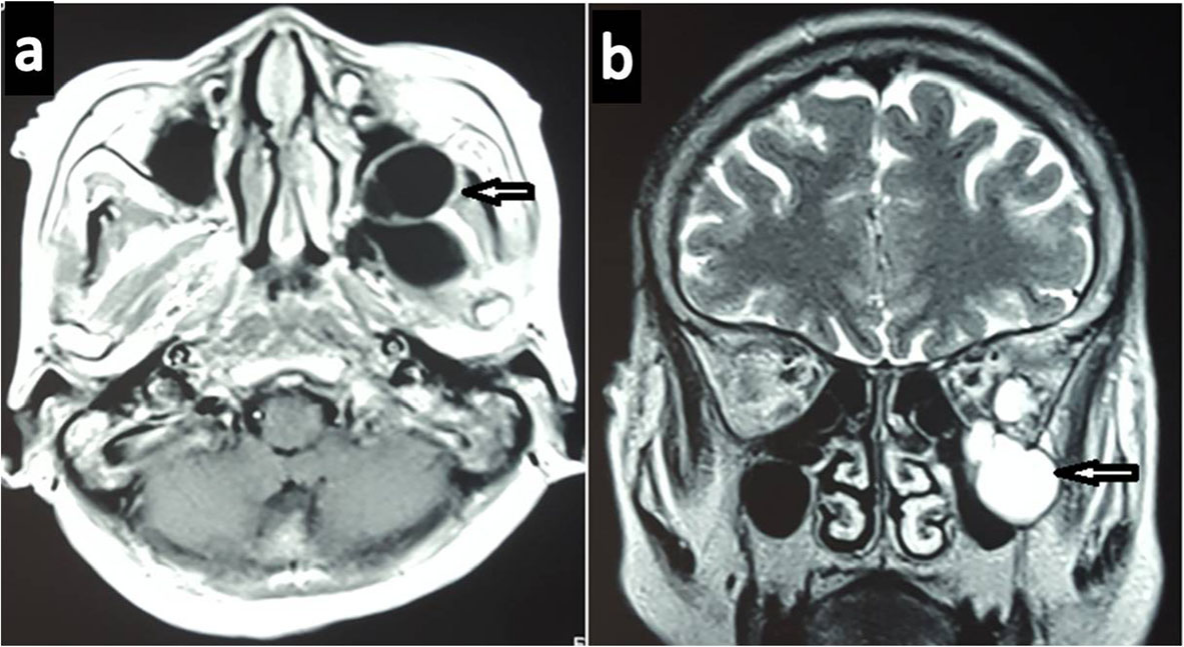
Axial T1-weighted (a) and coronal T2-weighted magnetic resonance images showing cystic lesion (*arrows*) within the infratemporal fossa (b)
